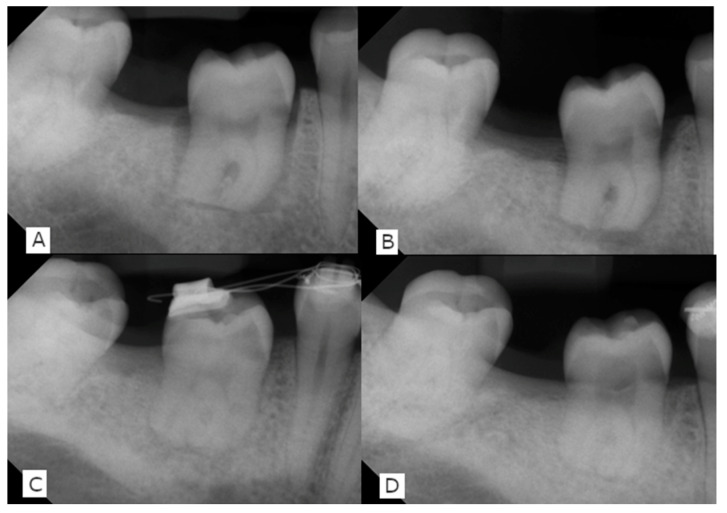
Radiographic follow-up with intraoral radiographs. (A) postop (B) 3 months postop beginning formation of the periodontal ligament space, no signs for external root resorption (C) 12 months postop, visible obliteration of the pulp chamber (D) 18 months postop radiolucent lesion in the pulp chamber.
